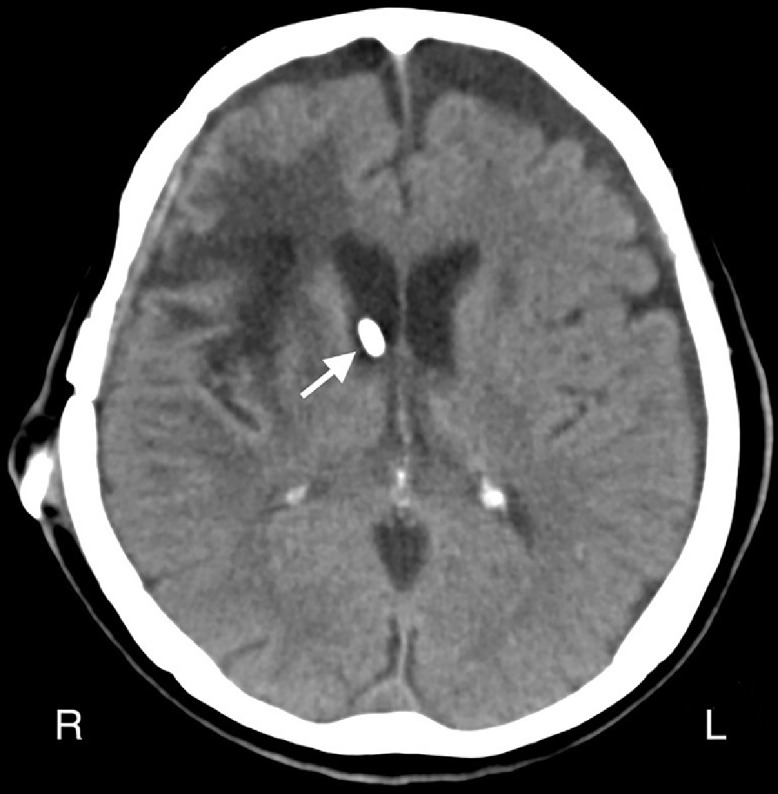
Head computed tomography scan taken on second admission to our hospital, on day 156 after the first ventriculoperitoneal shunt procedure, showing the tip of the ventricular tube (white arrow) in the right lateral ventricle.
